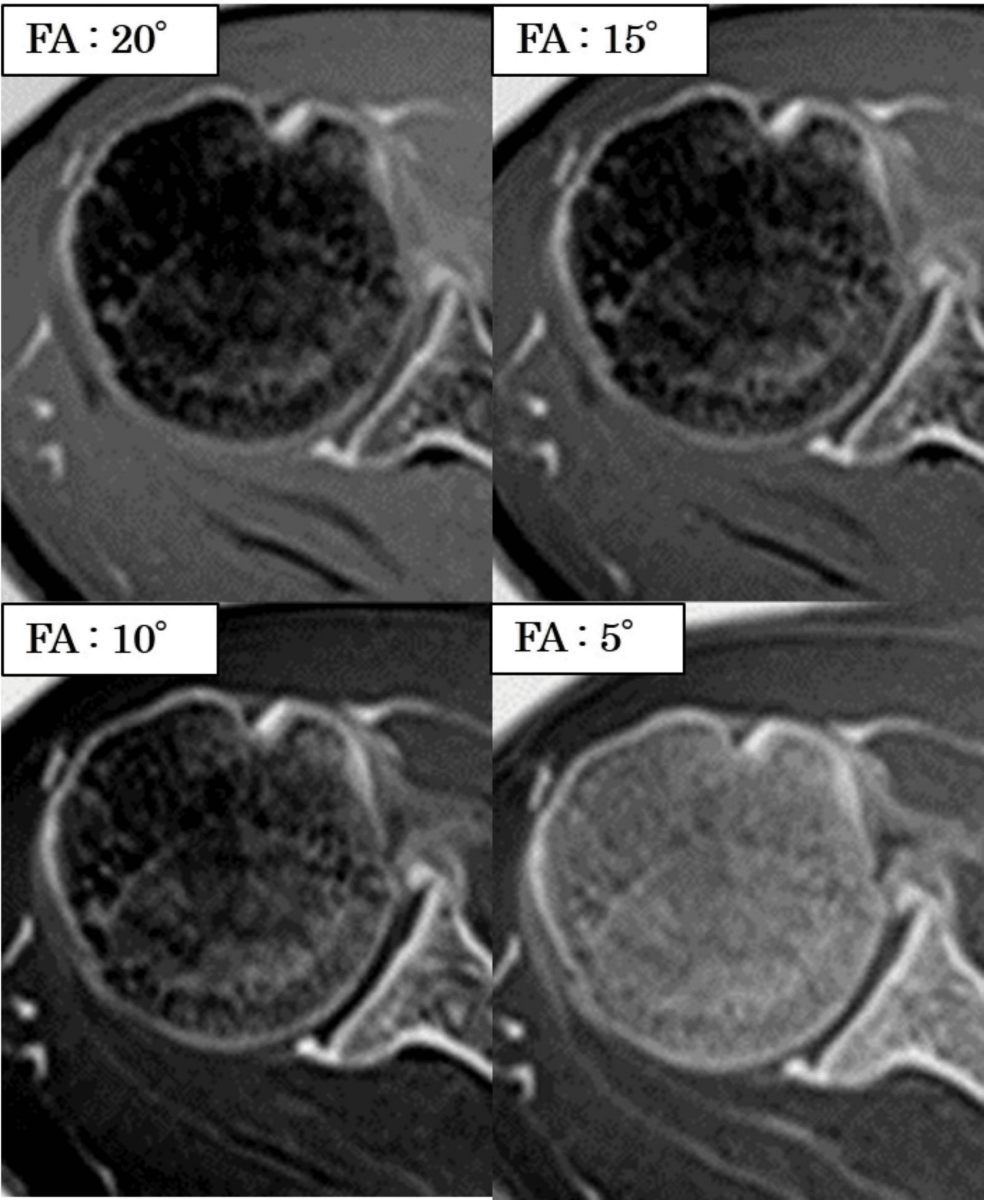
Signal inversion due to flip angle variations in grayscale-inverted CT-like MRI Representative images show the effect of flip angle on signal intensity in grayscale-inverted images. At a 5° flip angle, trabecular bone appears hypointense relative to muscle in the original image and appropriately hyperintense after inversion. At flip angles ≥10°, trabecular bone signal exceeds that of muscle before inversion, resulting in reduced contrast and potential misinterpretation in the inverted image. This highlights the importance of using lower flip angles to avoid signal inversion in fat-containing tissues.
